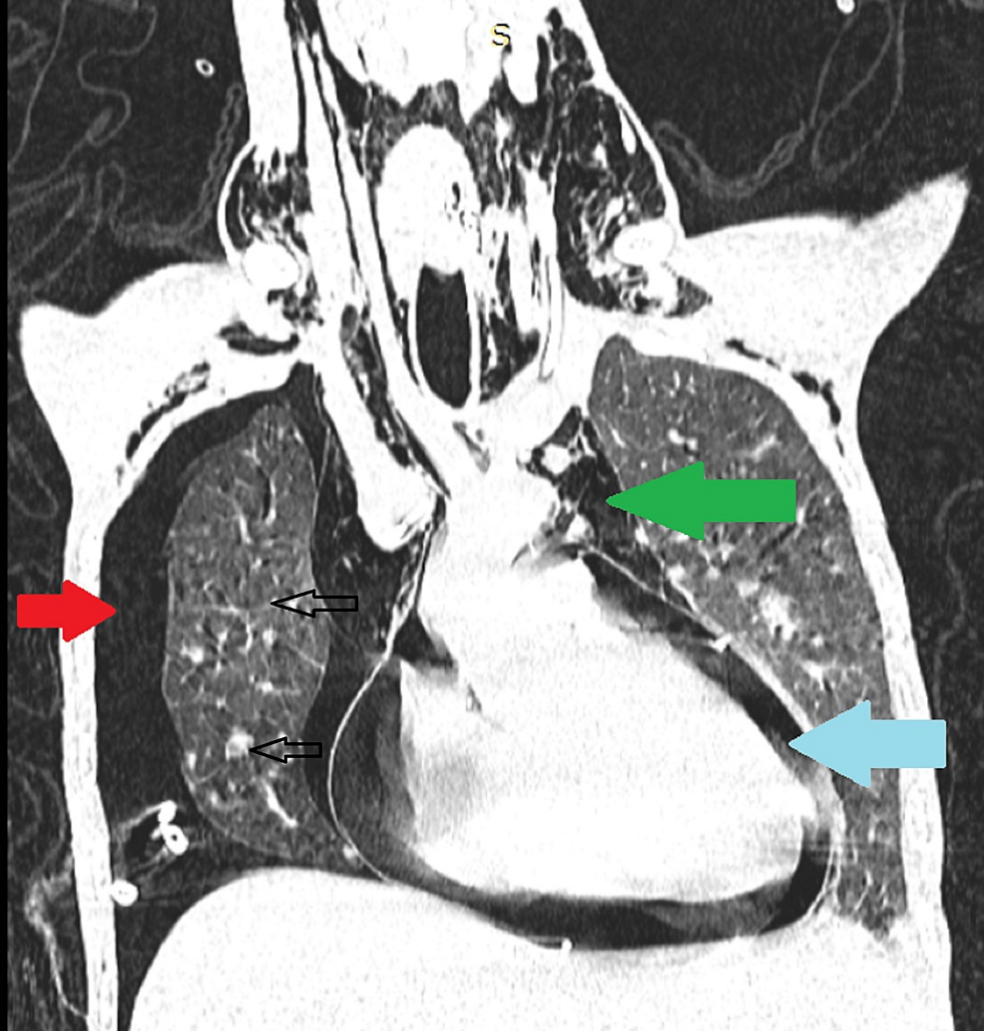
Contrast-enhanced computed tomography (CECT) chest showing the lung window of the patient. There is a presence of pneumothorax (red arrow), pneumomediastinum (green arrow), and pneumopericardium (blue arrow). Gross lung changes can be seen in the form of nodules and ground glassing in the background.
